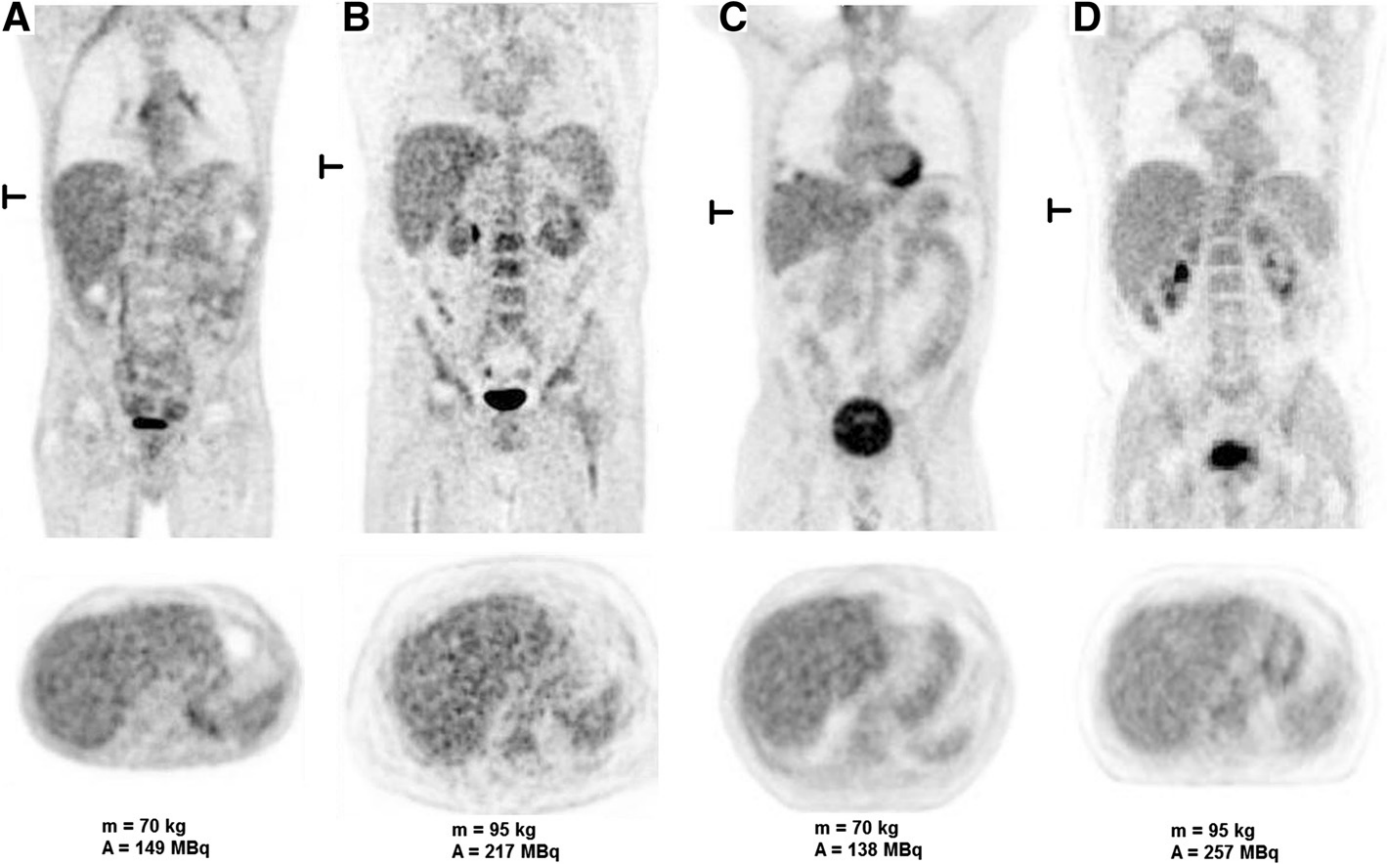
Patient scans in the old and new dose regimens. Coronal and transverse slices of a whole-body FDG-PET scan for (A) a patient of 70 kg (dose = 149 MBq) and (B) a patient of 95 kg (dose = 217 MBq), using the old dose regimen, and for (C) a patient of 70 kg (dose = 138 MBq) and (D) a patient of 95 kg (dose = 257 MBq), using a quadratic relation between body mass and FDG dose. Using the old relation between body mass and dose, the dose would have been (C) 149 and (D) 217 MBq, respectively. All four scans were performed on the Biograph TruePoint. The location of the transverse slice is indicated on the coronal view.
